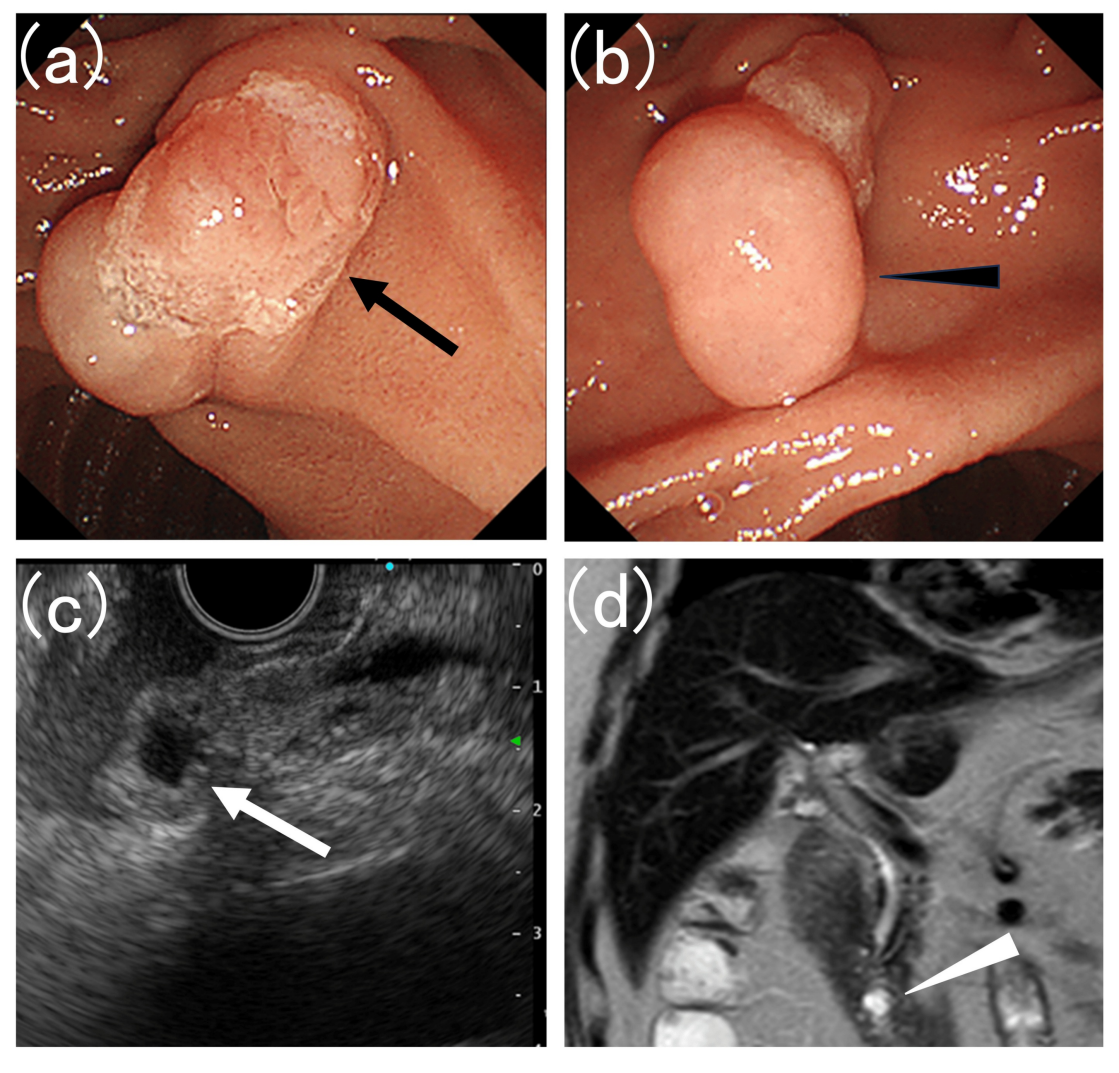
Images of an ampullary adenoma with cystic components (a) Endoscopic examination with a duodenoscope revealed a duodenal papillary tumor (black arrow), and biopsy confirmed the lesion as an adenoma. (b) The ampullary adenoma was associated with a submucosal mass extending toward the anal side (black arrowhead). (c) EUS showed the submucosal mass on the anal side of the ampullary adenoma to be internally anechoic, indicating a cystic nature (white arrow). (d) A coronal T2-weighted magnetic resonance image revealed that the submucosal mass contained only fluid components (white arrowhead), further suggesting it was a cyst. EUS, endoscopic ultrasound
